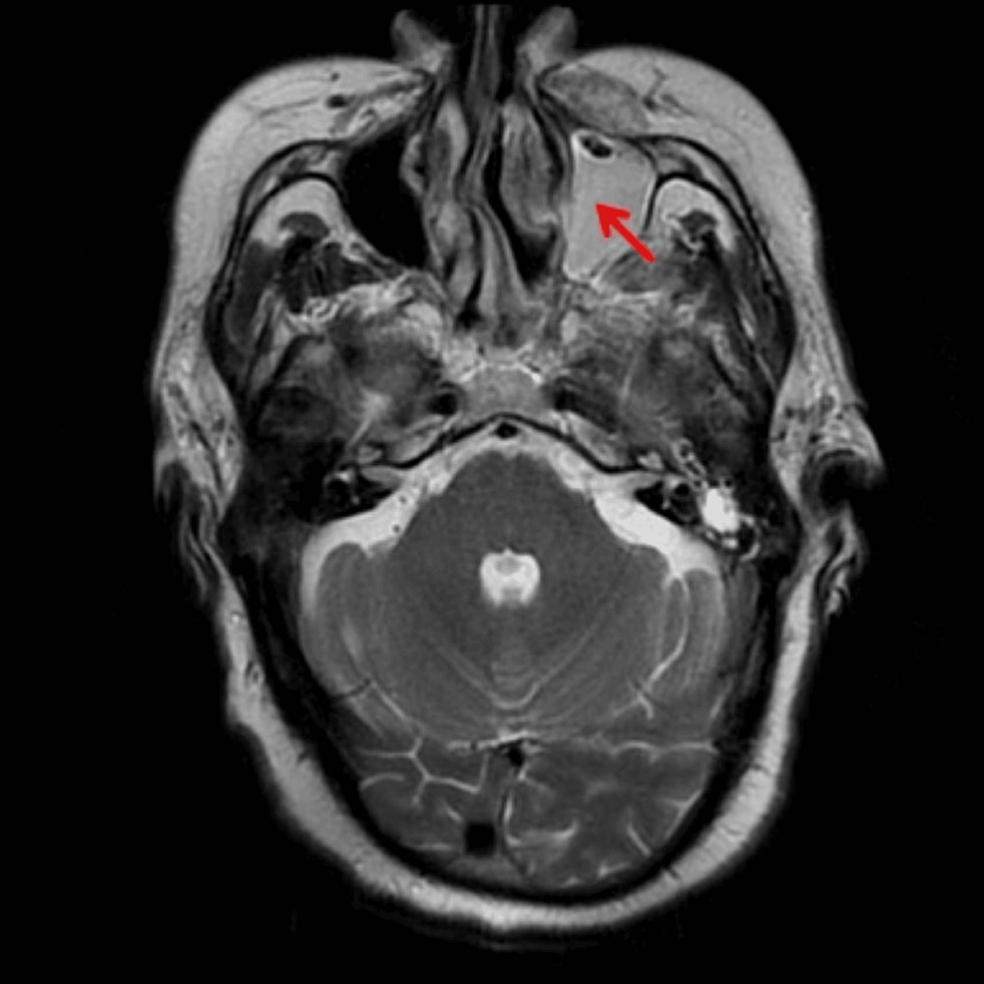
MRI (T1 image) reveals complete opacification of left maxillary sinus with homogenous high signal intensity (red arrow)
